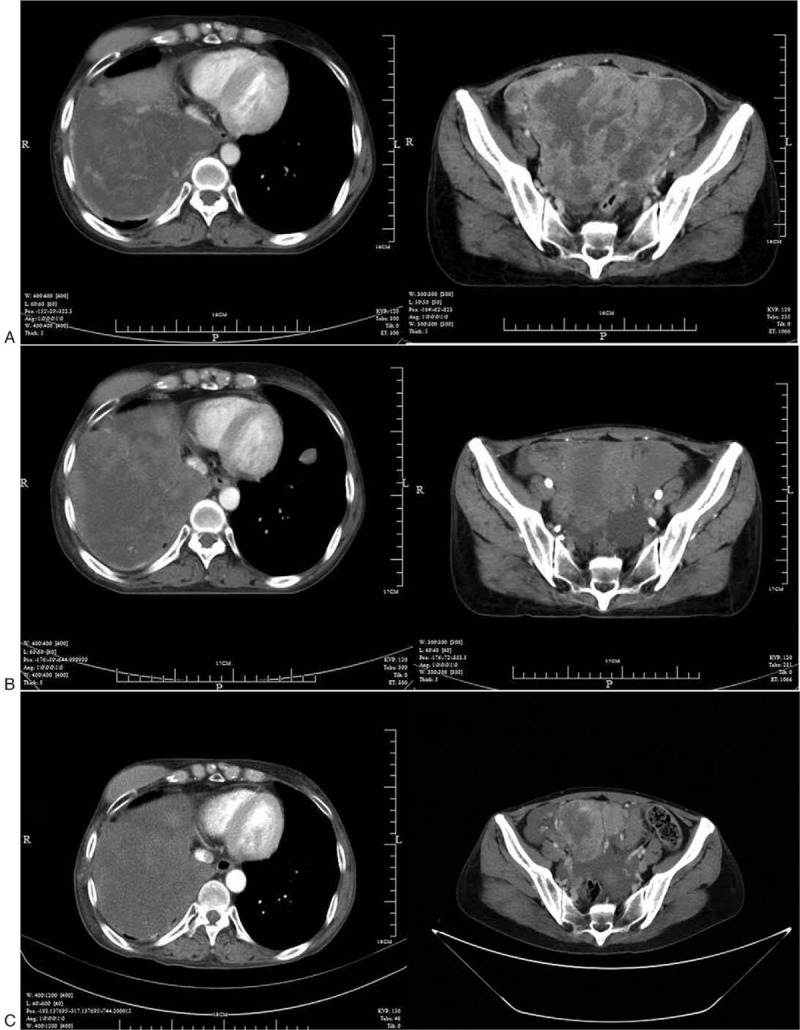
Computed tomography showed huge masses in the right lower chest and pelvis before taking apatinib (A); Computed tomography showed the size of mass following treatment with apatinib of 500 mg daily for 3 months (B); Computed tomography showed the size of mass after taking apatinib for 6 months (C).
